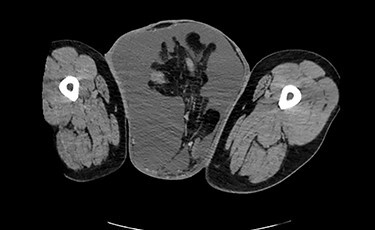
CT (axial view) demonstrating large left inguinal hernia sac containing bowel, fluid and left ureter.
